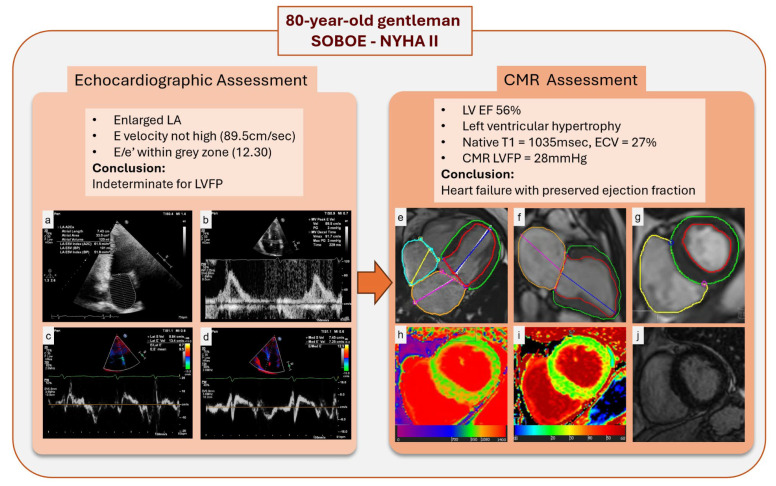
Multimodal assessment of LVFP and myocardial characteristics in an 80-year-old male with shortness of breath on exertion and NYHA class II symptoms. Panel (a–d): Echocardiographic assessment demonstrates an enlarged LA, an E velocity of 89.5 cm/sec, and an E/e’ ratio of 12.3, placing LVFP in an indeterminate range. Panel (e–g): CMR showed left ventricular hypertrophy and preserved LVEF, similar to echocardiography. (h,i): Native T1 mapping and extracellular volume (ECV) fraction mapping reveal regions of increased values, suggesting subtle myocardial fibrosis. (j): Late gadolinium enhancement (LGE) imaging shows no noticeable scar. Abbreviations: SOBOE, shortness of breath on exertion; NYHA, New York Heart Association; LA, left atrium; LVFP, left ventricular filling pressure; LVEF, left ventricular ejection fraction; CMR, cardiac magnetic resonance; ECV, extracellular volume; LGE, late gadolinium enhancement.
